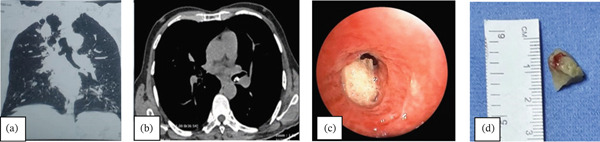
(a and b) Chest CT showing radio‐opaque FB in left main stem bronchus. (c) Pale‐yellow FB in left main bronchus with bony appearance. (d) Retrieved chicken bone.
